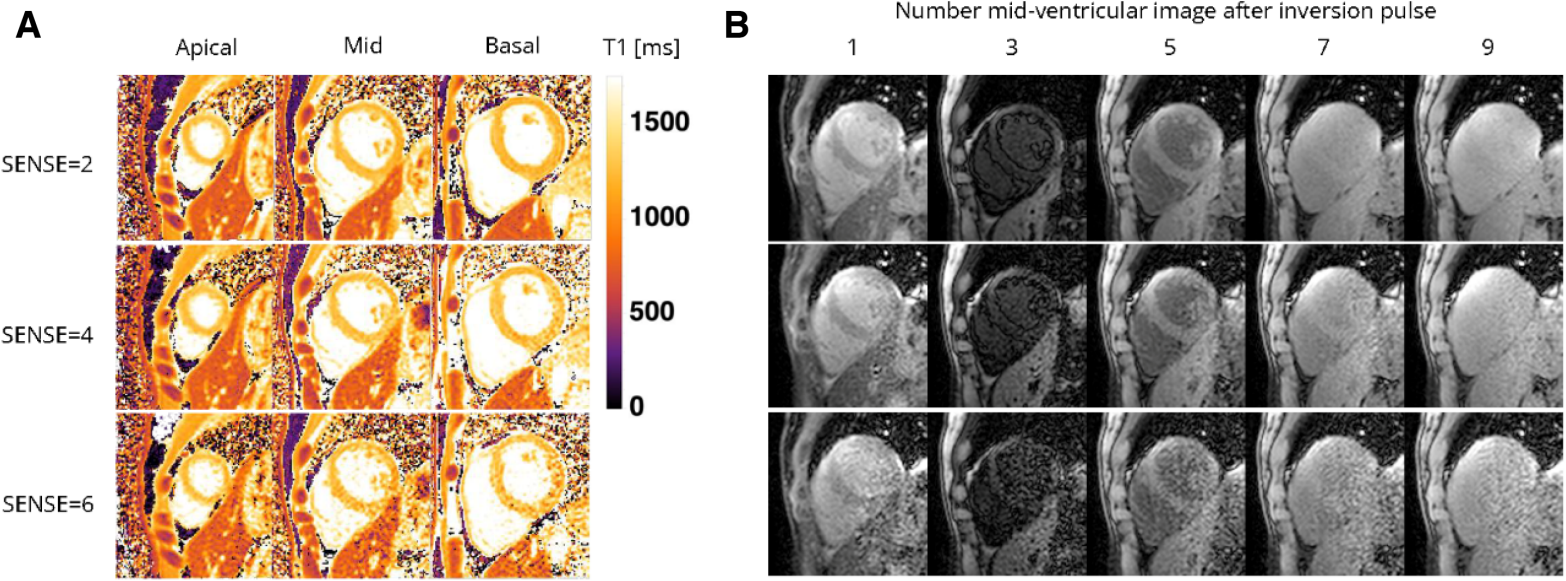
Representative (A) T1 maps and (B) T1-weighted images (time points 1, 3, 5, and 7 in an inversion train of 10) of a healthy female volunteer acquired with the 72-channel array coil using the single BH shared-inversion pulse interleaved MOLLI with SENSE = 2 and 6. Lighter colors indicate higher T1 values and darker colors indicate lower T1 values according to the color bar. ms, milliseconds.
